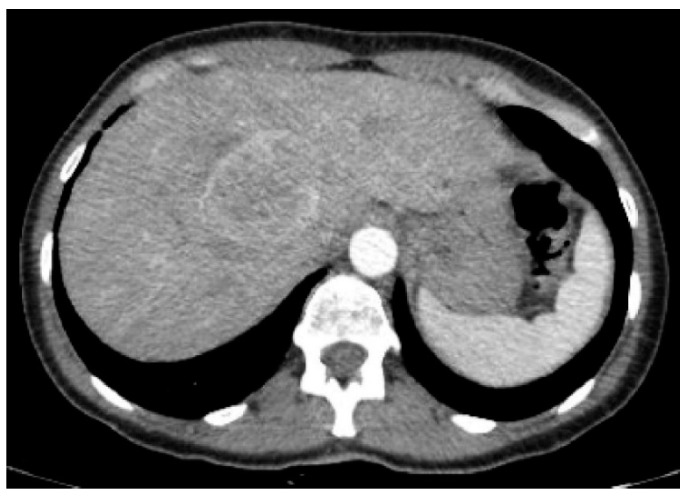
Computed tomography shows tumor with perivascular epithelioid cell tumor with strong contrast enhancement in the arterial phase.
